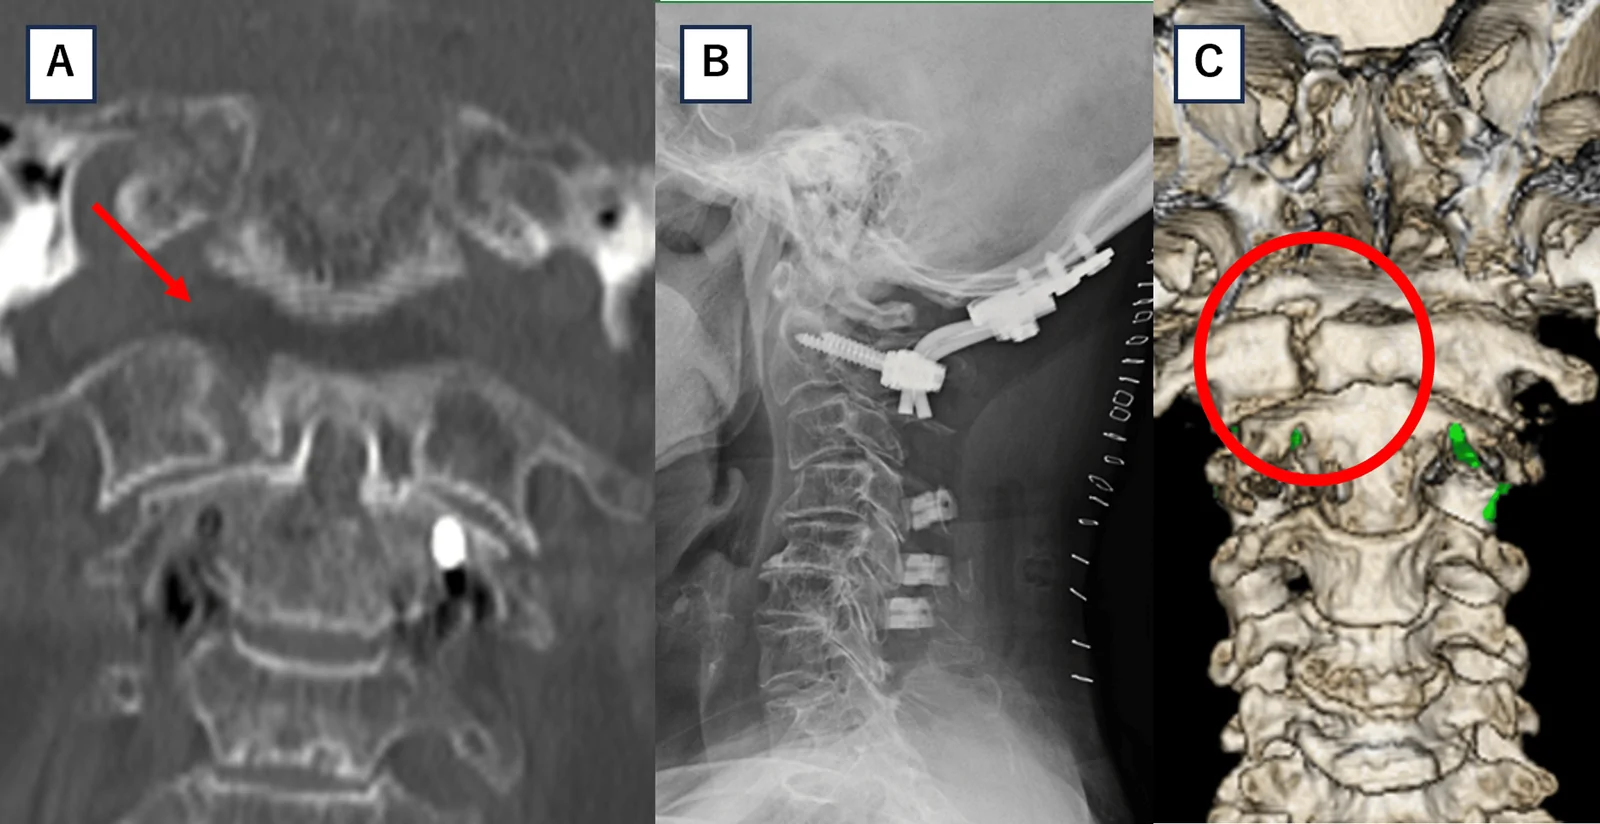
Postoperative imaging after occiput-to-C2 fixation (A) Coronal CT image after fixation. (B) Sagittal radiograph demonstrating restored alignment. (C) Three-dimensional reconstructed CT image after occiput-to-C2 fixation CT: computed tomography
